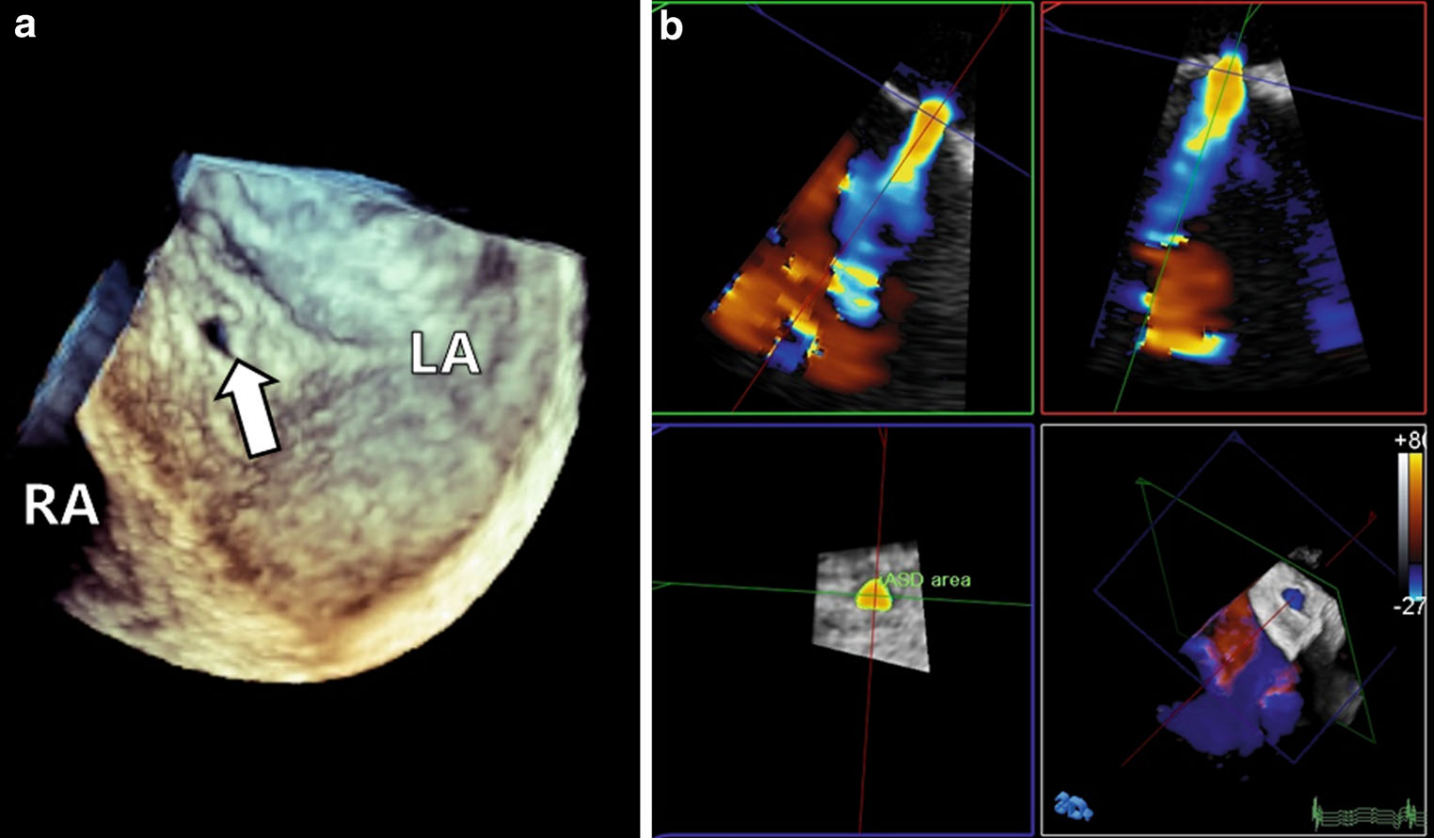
3D view of iatrogenic atrial septum defect (a) and display of shunt using colour Doppler (b)
